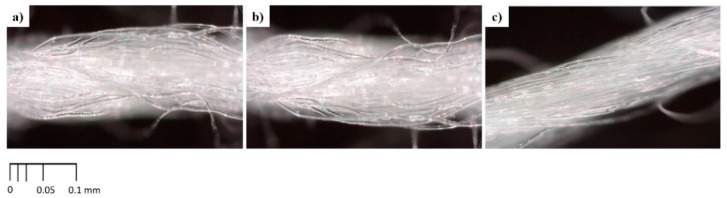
Uncoated cotton thread surface of (a) CT-A, (b) CT-B, and (c) CT-C.
